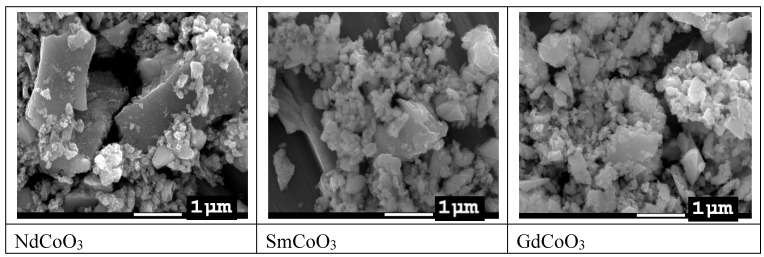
SEM images of nanostructured samples after ultrasonic treatment.
